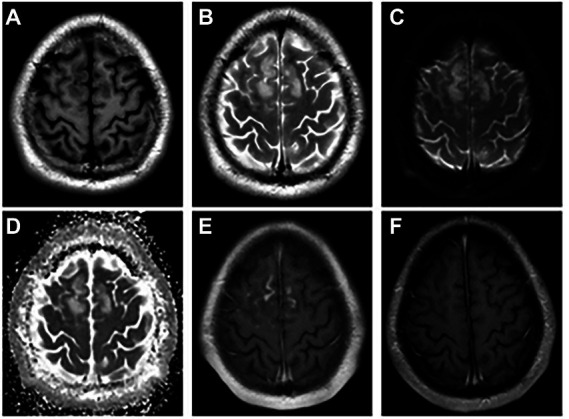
The abnormal MRI was obtained from patient 1. A 21-year-old woman with serum and CSF MOG antibodies, presenting with low-to-moderate fever, seizures, headache, transient motor aphasia, and bilateral palsy of two legs. Bilateral frontal–parietal falciparum cortex are swollen, axis T1-weighted (A) and T2-weighted images (B) show significant signal alterations in bilateral frontal–parietal cortex and juxta cortical white matter. DWI (C) and ADC image show hyperintense signal (D), and enhancement the corresponding of lesions are enhanced (E). Re-examination MRI enhancement (F) at discharge shows enhanced lesions disappeared. DWI = Diffusion Weighted Imaging, ADC = apparent diffusion coefficient, MOG = myelin oligodendrocyte glycoprotein, CSF = cerebrospinal fluid.
